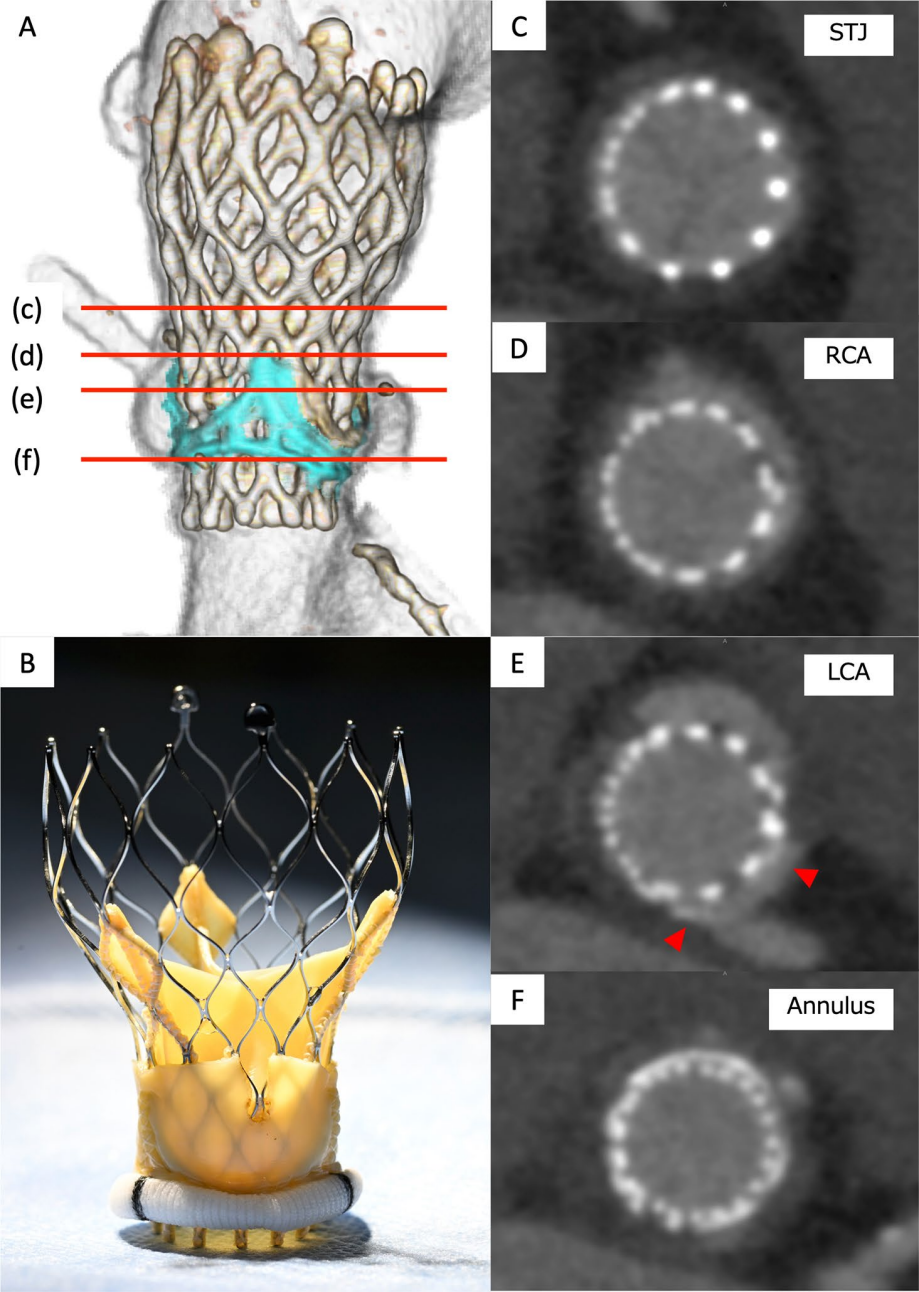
Post-procedural multidetector computed tomography images. a Volume-rendering image of the aortic root showing 23-mm Evolut pro plus (white) and 19-mm Trifecta (light blue). b Corresponding in vitro image demonstrating that a lacerated leaflet creates communication between the native left sinus of Valsalva and neo-sinus. c An axial image of the Sino-tubular junction shows the waist of the Evolut contacts circumferentially to the aorta. d, e Axial images at the level of right and left coronary arteries demonstrate contrast enhancement of both right and left sinuses of Valsalva, whereas non-coronary sinus is not enhanced. Inside the left cups, a calcified left leaflet of Trifecta separates on both sides (red arrow heads). f An annuls level image showed Evolut expanding to a circle with a diameter of 14.5 mm
